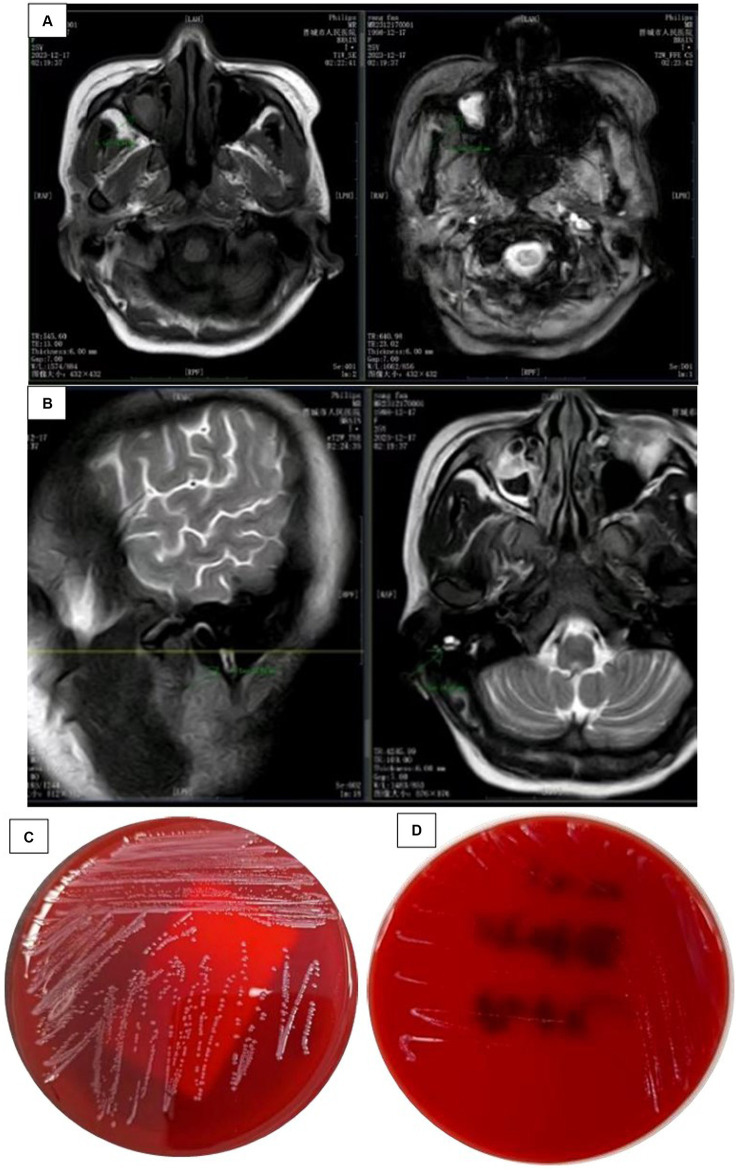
(A) Head MRI findings: a plain scan of the head MRI shows obvious abnormalities, with rig maxillary sinusitis (excluding fungal lesions) and (B) Head MRI findings: a plain scan of the hea MRI shows significant abnormalities, with MR changes in right mastoiditis. (C) Cultured CSI colonies and (D) the growth of bacterial colonies in the refrigerator.
